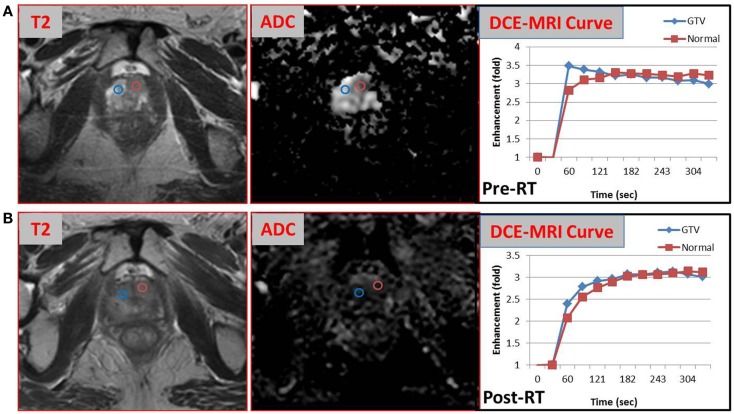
Comparison of MP-MRI exam before and after radiotherapy. T2-weighted MRI, ADC map, and contrast-versus-time curves pre- (A) and 3 months post-RT (B) of two prostate regions are shown: malignant lesion pre-treatment (blue) and healthy appearing PZ (red).
